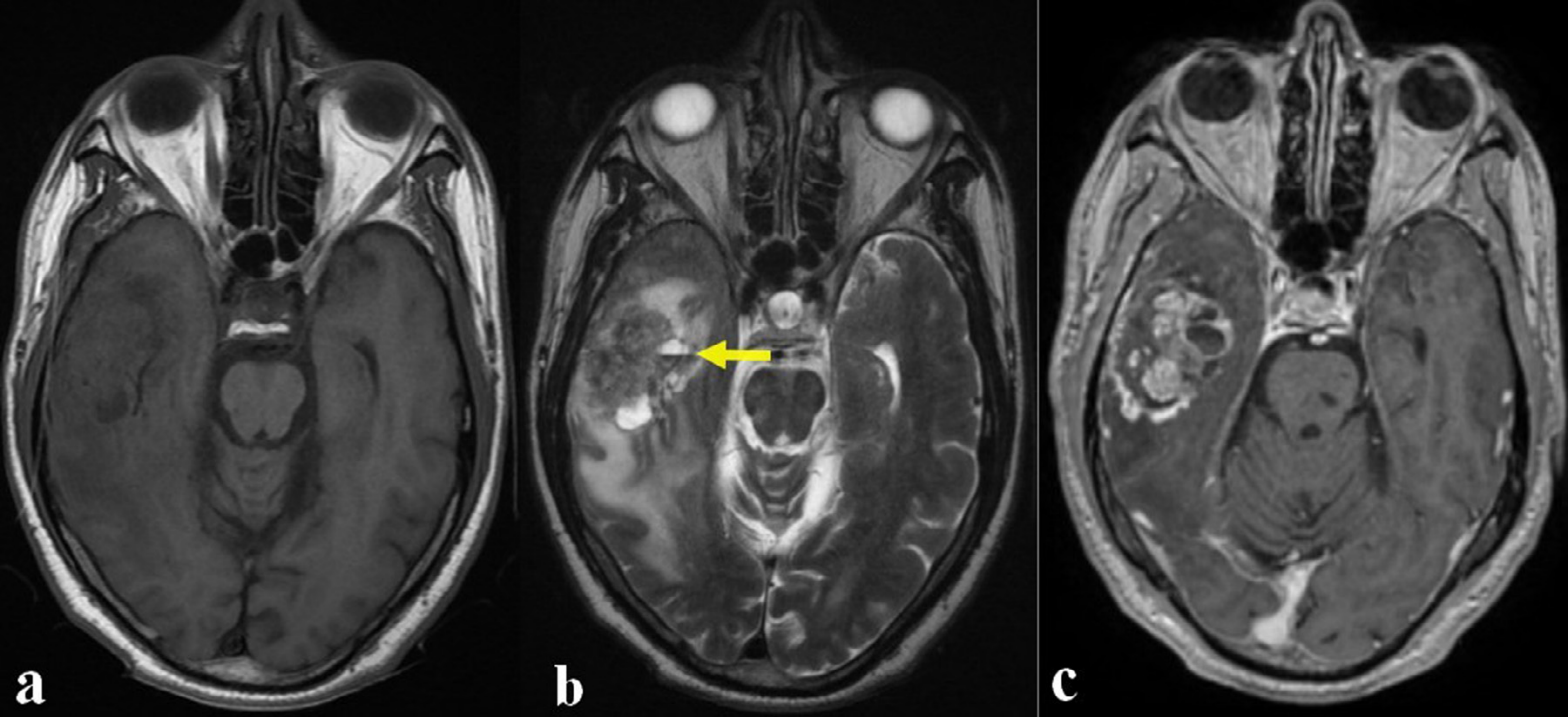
Brain MRI in axial plane showing a right temporal tumor, in solid and cystic geographical map of polycyclic contours. Its fleshy portion had an isosignal on T1-weighted sequences (a) and a heterogeneous signal on the T2-weighted sequences (b). This lesion developed areas of necrosis and is the seat of a liquid liquid level (blood liquid) (b; yellow arrow). Gadolinuim chelate enhancement was heterogeneous (c).
